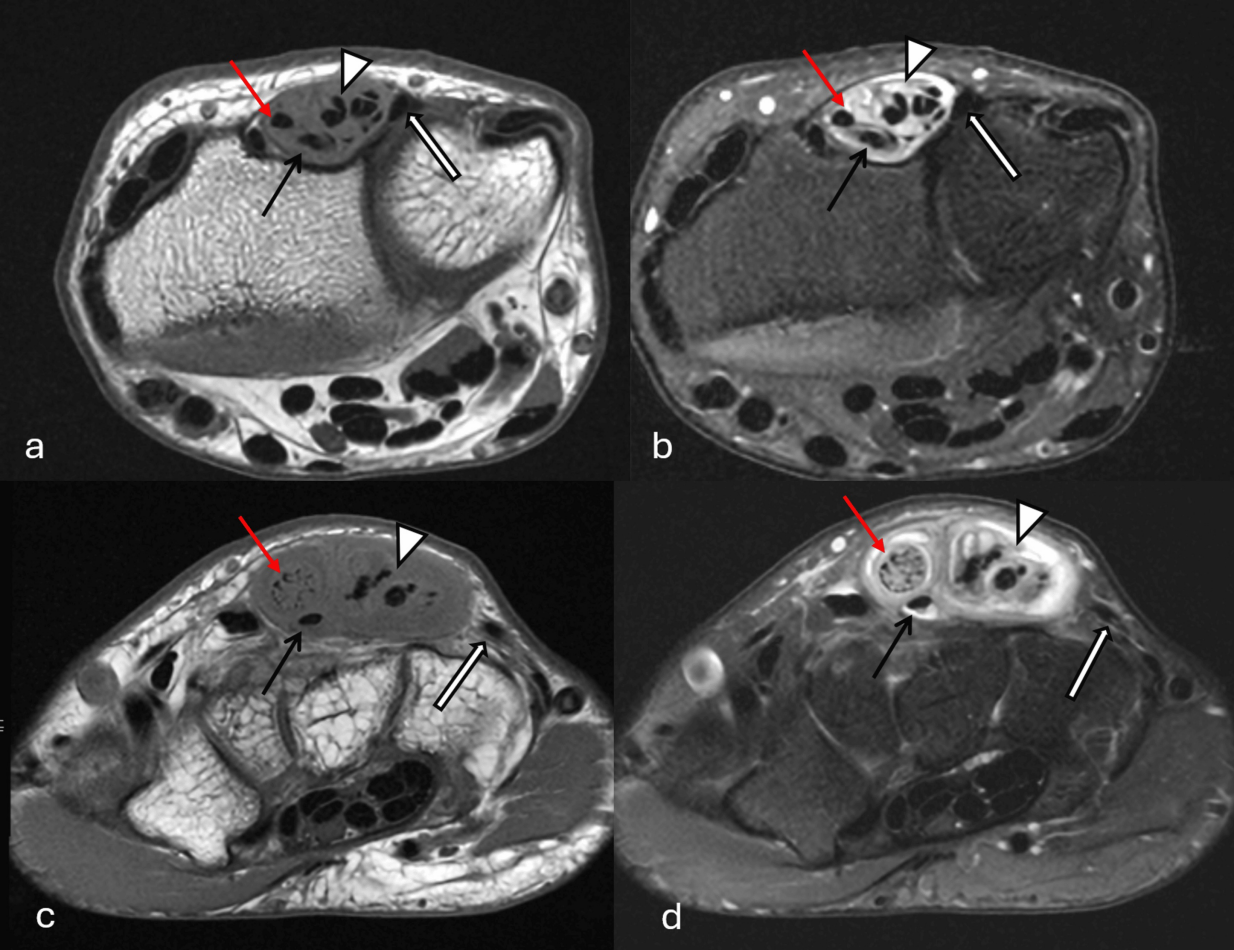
T1W and T2W fat-suppressed images of MRI wrist T1-weighted (T1W) axial images (a, c) and T2-weighted (T2W) fat-suppressed images (b, d) of the MRI right wrist of the patient. They demonstrated grossly thickened extensor digitorum tendons with moderate-volume sheath fluid and sheath thickening. Heterogeneous para- and intratendinous signal changes were observed, compatible with tenosynovitis and gouty tophus infiltration. Note the significant tendon thickening at the extensor digitorum communis tendons to the second digit (red arrows), thickened extensor digitorum communis tendons to the third, fourth, and fifth digits with suspect longitudinal tears (arrowheads). Preserved extensor indicis tendon (black arrows) and extensor digiti minimi tendon (block arrows). MRI provided a detailed visualization of the anatomy. The MRI results were consistent with the intraoperative findings MRI: magnetic resonance imaging
